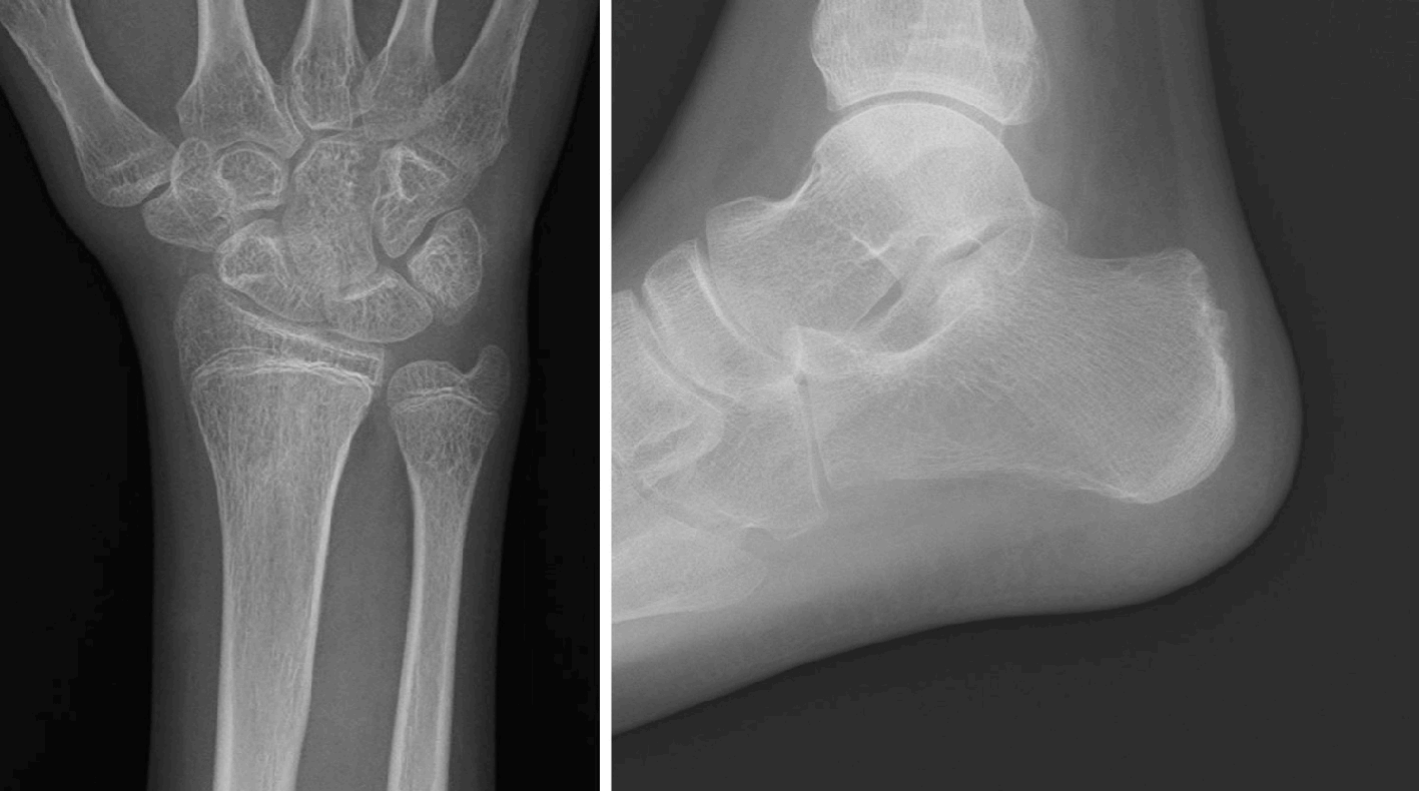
Radiographs obtained at the final follow-up, 3 years after the initial visit. No significant skeletal deformities or long-term complications were observed.
